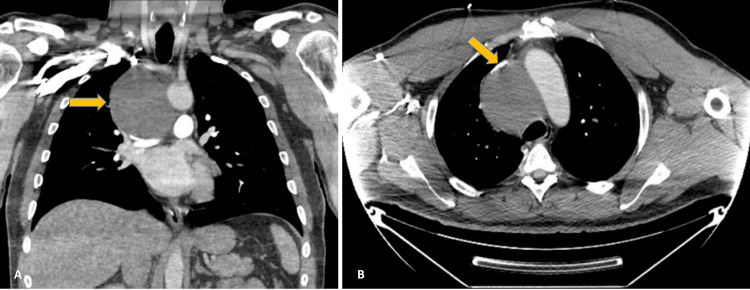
(A) CT scan of the chest with homogenous soft-tissue mass in the mediastinum. (B) Mass causing stenosis of the SVC with compression of the distal trachea and carina
